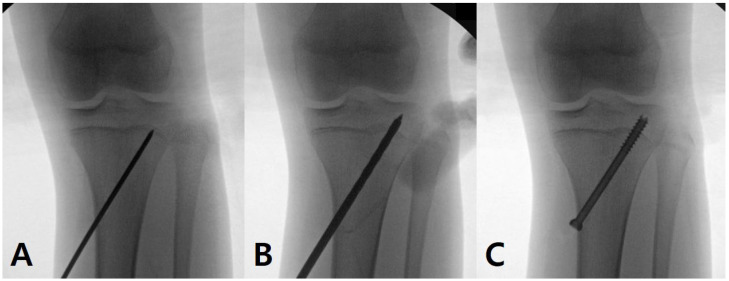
Hemi-percutaneous epiphysiodesis using a transphyseal screw at the lateral portion of the proximal tibial physis. A 6.5 mm cannulated screw was inserted through a 1.0 cm skin incision from medial proximal metaphysis. The lateral one fourth of the physis was targeted using a guide pin. To minimize the physeal damage, the guide pin entered the epiphysis after confirming its position by intraoperative radiographs (A). During drilling, continuous saline irrigation was performed and a cannulated drill bit repeatedly changed into the other one to minimize the thermal damage (B). The final screw position had enough threads (at least three) on both the below and top of the physis (C).
